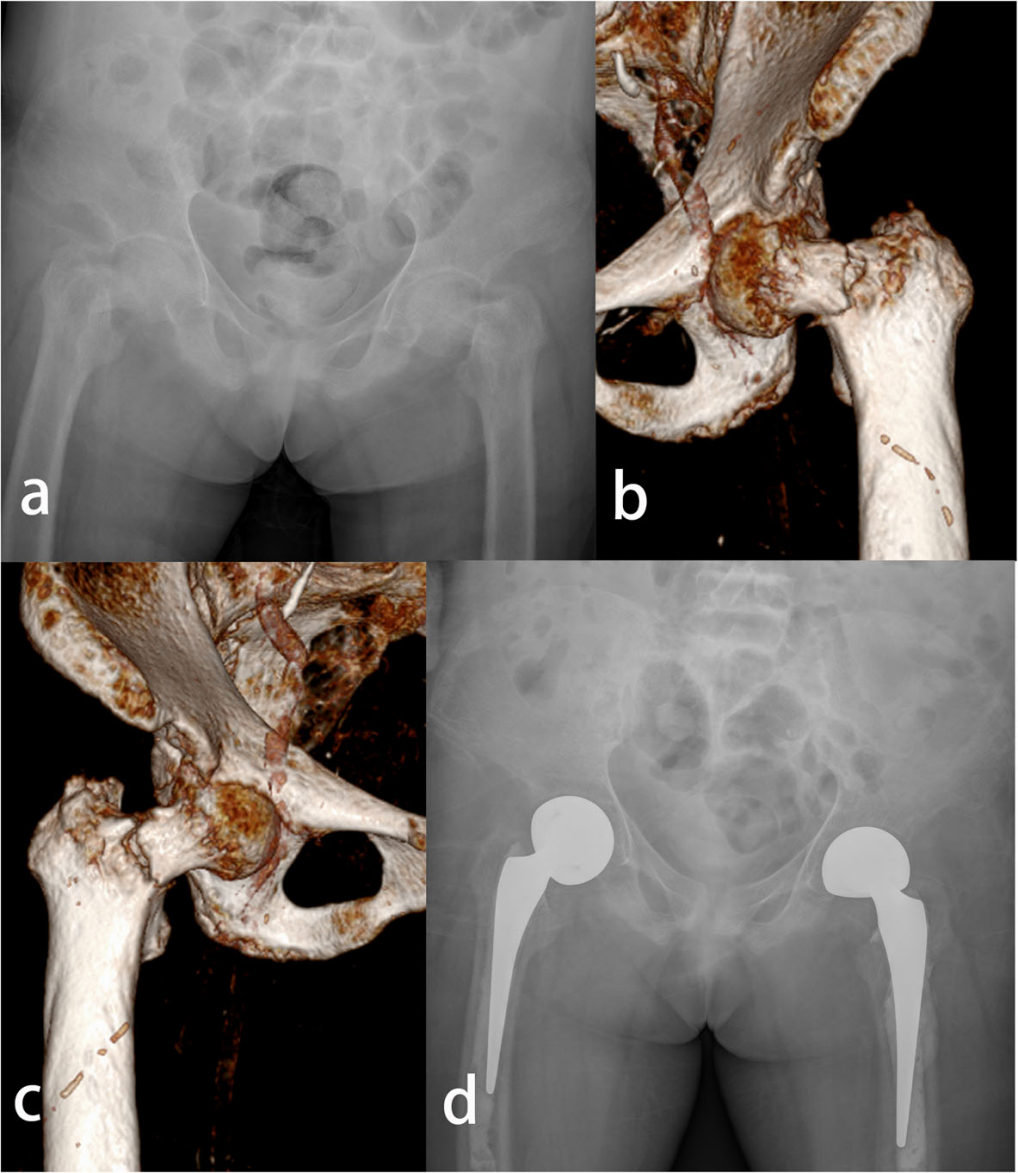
a: Hip X-ray showing bilateral femoral displaced fractures with low bone density. b: CT scan showing the femoral displaced fracture on the left side. c: CT scan showing the femoral displaced fracture on the right side. d: Bilateral femoral displaced fractures treated with bilateral hip hemiarthroplasty
